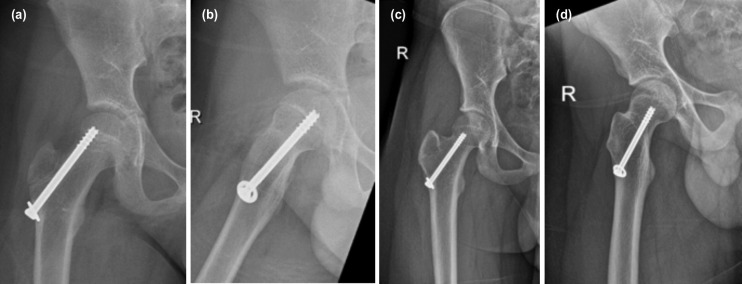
(a, b) Plain radiographs showing the healed fracture one year after surgery. (c, d) Plain radiographs five years after surgery showing a normal right hip.
